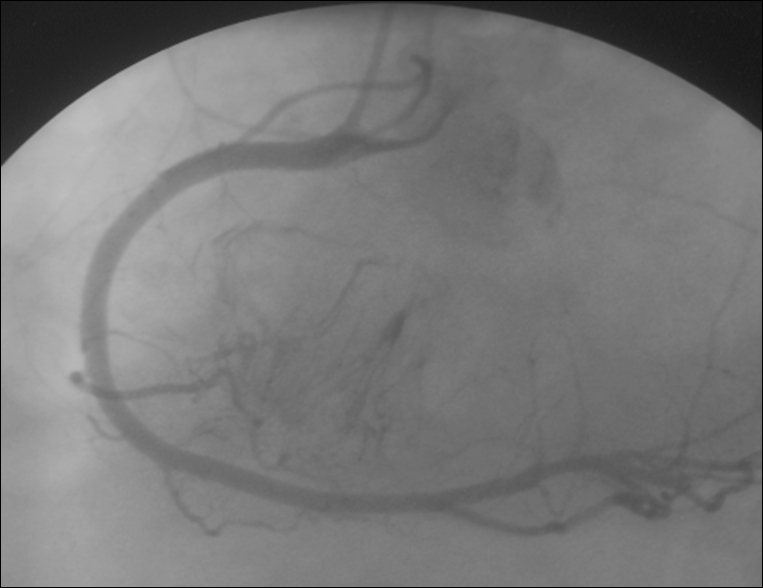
Computed tomography scan showing the presence of a right heart tumor developing on both sides of the tricuspid valve.
